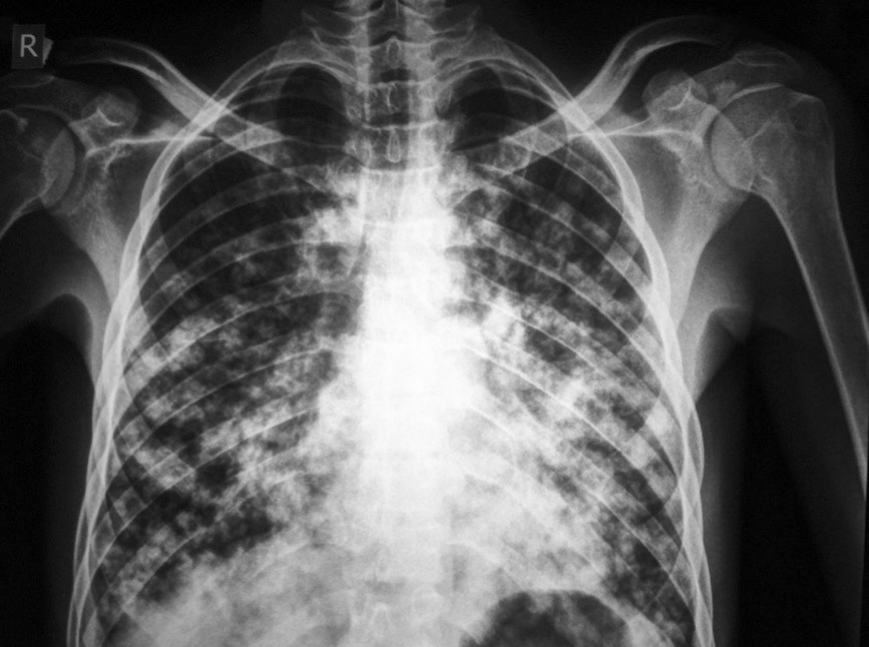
Chest X-ray showing multiple bilateral lungs patches with the differential diagnosis of secondaries, miliary tuberculosis, or mycetoma infiltrations.
